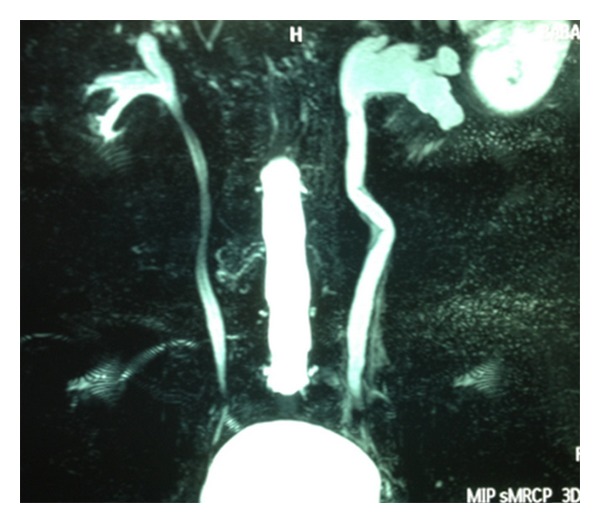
Coronal MR image demonstrating left-sided hydroureteronephrosis.
